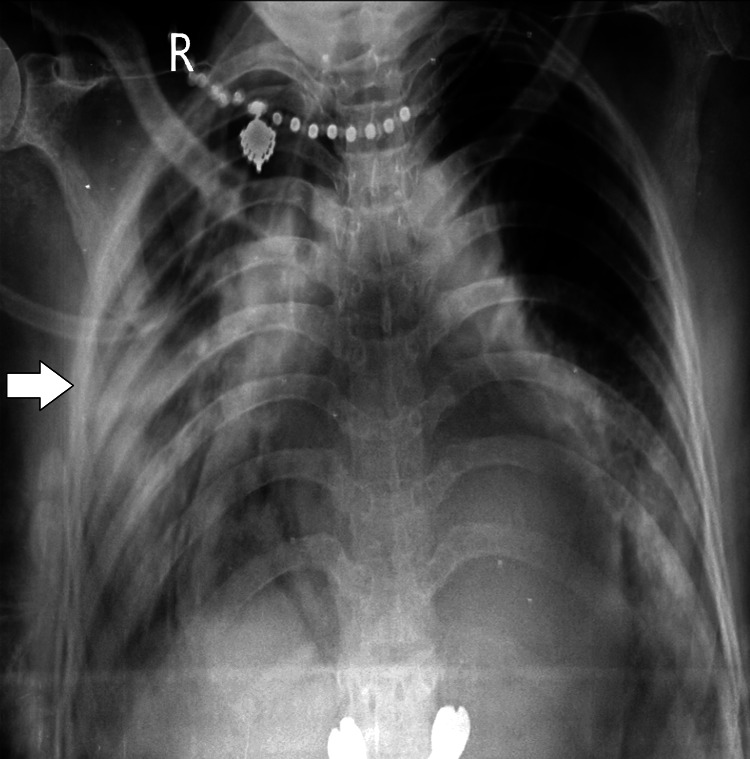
Chest X-ray posteroanterior (PA) view The image reveals consolidation in the right middle and lower lobes, as well as consolidation in the left lower lobe of the lung
